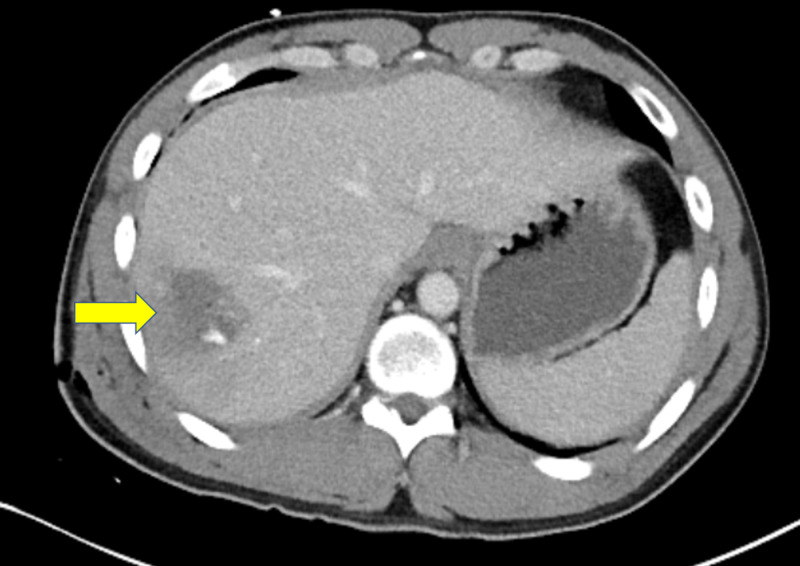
Abdomen CT, axial view, showing grade 3 hepatic laceration from knife stab wound (yellow arrow)
